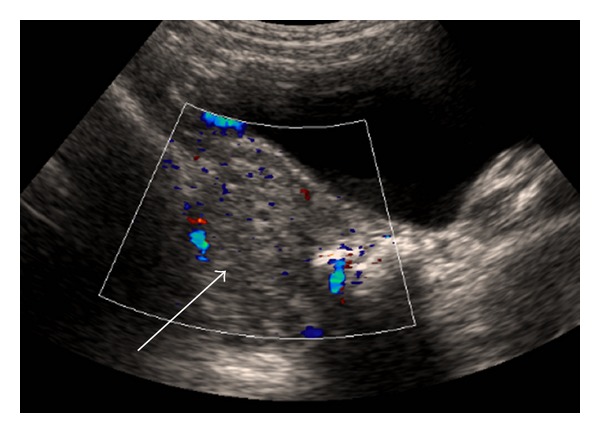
Doppler ultrasound of a large right ovarian cyst (estimated ovarian volume of 41 cm^3^) demonstrating arterial flow but scant venous outflow.
